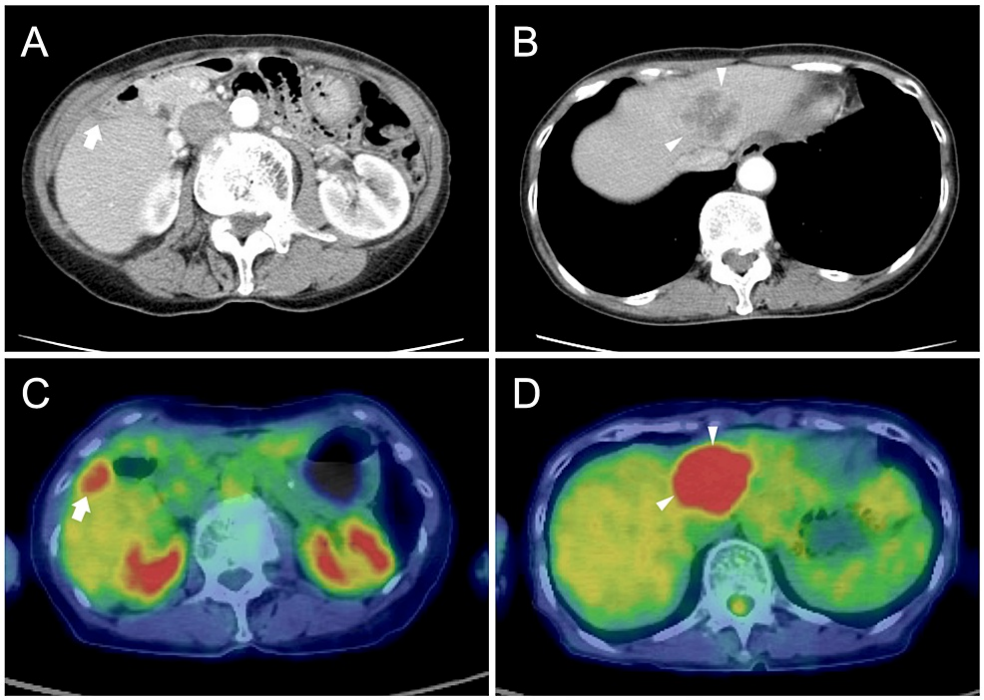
Diagnostic images during metachronous liver recurrence diagnosis. Contrast-enhanced CT shows a hypovascular tumor (arrow) in segment V of the liver surface (A) and a hypovascular tumor (arrowheads) with peripheral enhancement in segment IV (B). PET/CT shows the maximum standard uptake value (SUVmax) at the segment V lesion was 3.87 (C, arrow) and the SUVmax at the segment IV lesion was 12.5 (D, arrowheads).
